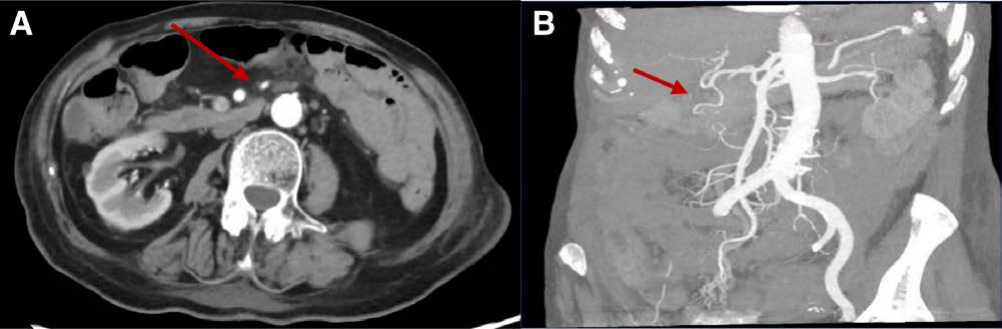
Abdominal CTA shows the superior mesenteric (A) and right renal artery artery (B) with mild atherosclerosis (arrows). CTA = computed tomography angiogram.
